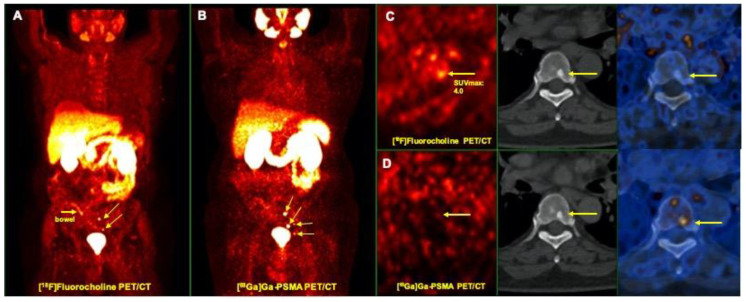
Prostate cancer recurrence: small skeletal metastases exclusively detected by [^18^F]Fluorocholine PET/CT. (A) [^18^F]Fluorocholine PET: MIP shows two small lymph nodes on the left pelvis suggestive of lymph node metastases (arrows). (B) [^68^Ga]Ga-PSMA PET/CT: MIP shows multiple intensive [^68^Ga]Ga-PSMA positive pelvic lymph nodes, clearly superior to [^18^F]Fluorocholine PET/CT (arrows). (C) [^18^F]Fluorocholine PET/CT (transaxial PET (left), CT (middle) and fusion PET/CT (right) images) exclusively detected a single bone metastasis on T6 vertebra well correlating with suspicious sclerosis on CT (arrows). (D) [^68^Ga]Ga-PSMA PET/CT (transaxial PET (left), CT (middle) and fusion PET/CT (right) images) shows limited value for detecting bone metastasis on T6 vertebra in spite of its superiority for detecting lymph node metastases.
